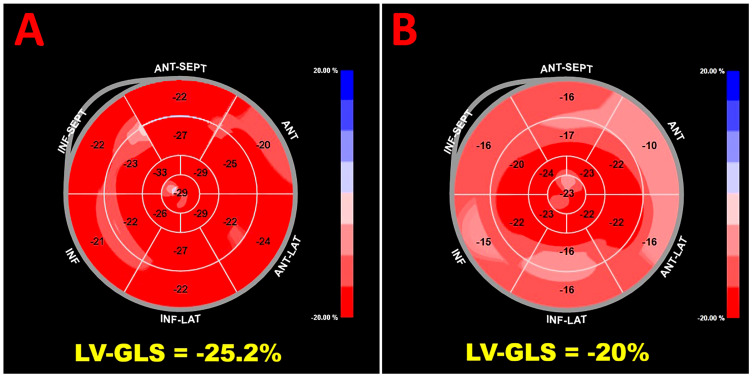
Speckle-tracking echocardiography-derived bull’s-eye plots of LV-GLS obtained in an Asian (A) and a Caucasian (B) pregnant woman enrolled in the present study. The more intense red color observed in the Asian pregnant woman reflects supranormal longitudinal left ventricular deformation, whereas the lighter red color in the European pregnant woman is mainly attributable to relatively reduced strain in the basal myocardial segments. LV-GLS, left ventricular global longitudinal strain.
